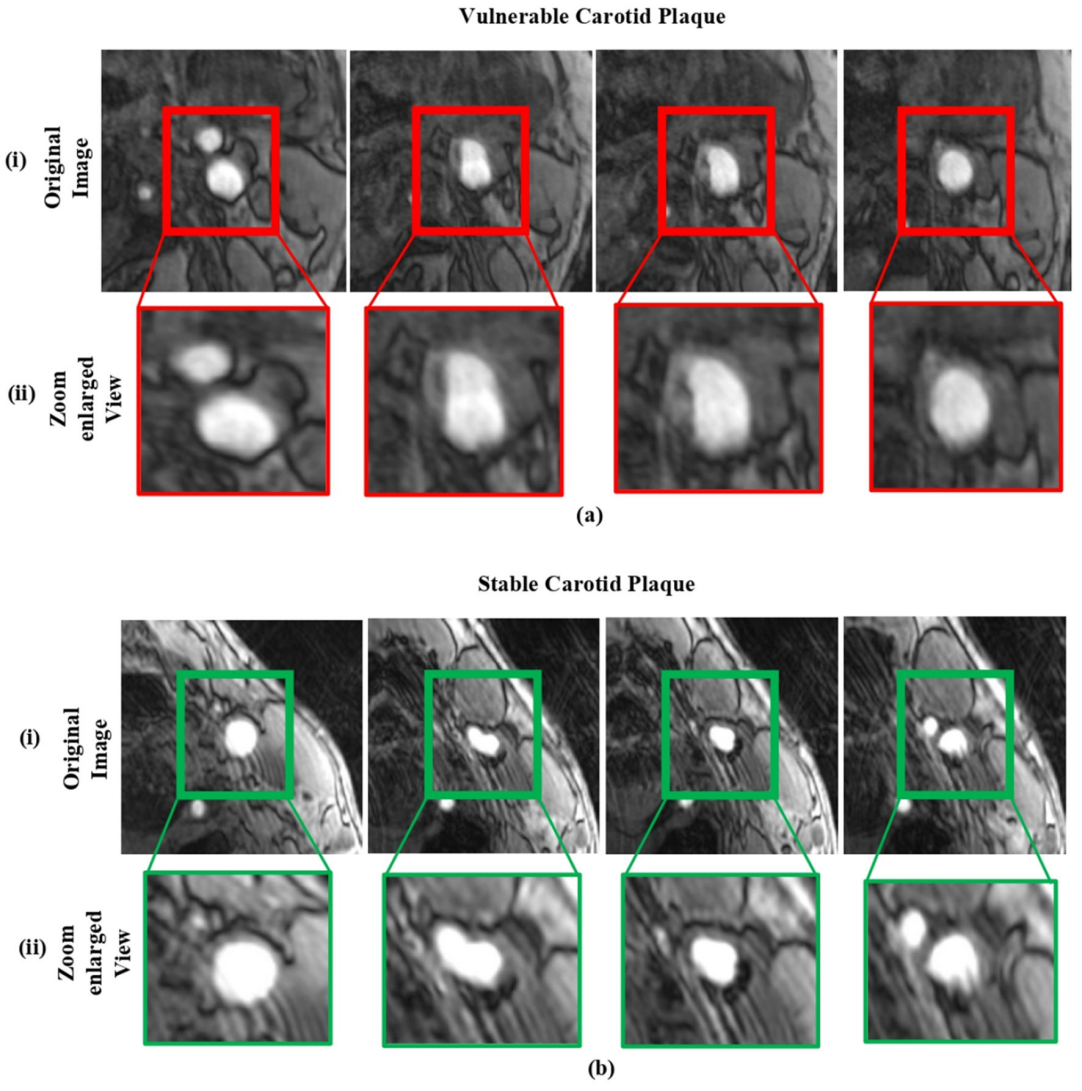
The sample dataset: (a) includes magnetic resonance images of vulnerable carotid plaques, and (b) includes images of stable carotid plaques. In each case, (i) shows the original magnetic resonance images, while (ii) displays their corresponding enlarged views.
